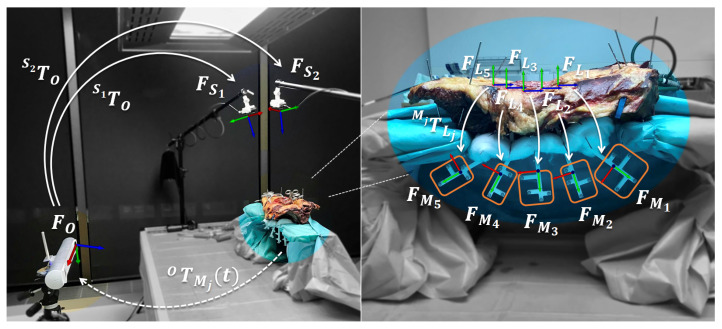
Setup overview with coordinate frames (*F*) and transformations (*T*). Dedicated markers ($F_{M_{1}},F_{M_{2}},\ldots ,F_{M_{5}}$) are attached to spine levels L1–L5 ($F_{L_{1}},F_{L_{2}},\ldots ,F_{L_{5}}$), enabling individual tracking (${}^{O}T_{M_{j}}\left(t\right)$) by the optical tracking system (OTS; $F_{O}$). The surgical site is viewed from the top by two RGB-D sensors ($F_{S_{1}},F_{S_{2}}$). Extrinsic calibrations between OTS and RGB-D sensors (${}^{S_{1}}T_{O},{}^{S_{2}}T_{O}$) can be found intraoperatively using the method described in Section 2.3. The relative marker poses w.r.t. the anatomy (${}^{M_{j}}T_{L_{j}}$) are determined in a postoperative CT (Section 2.6).
